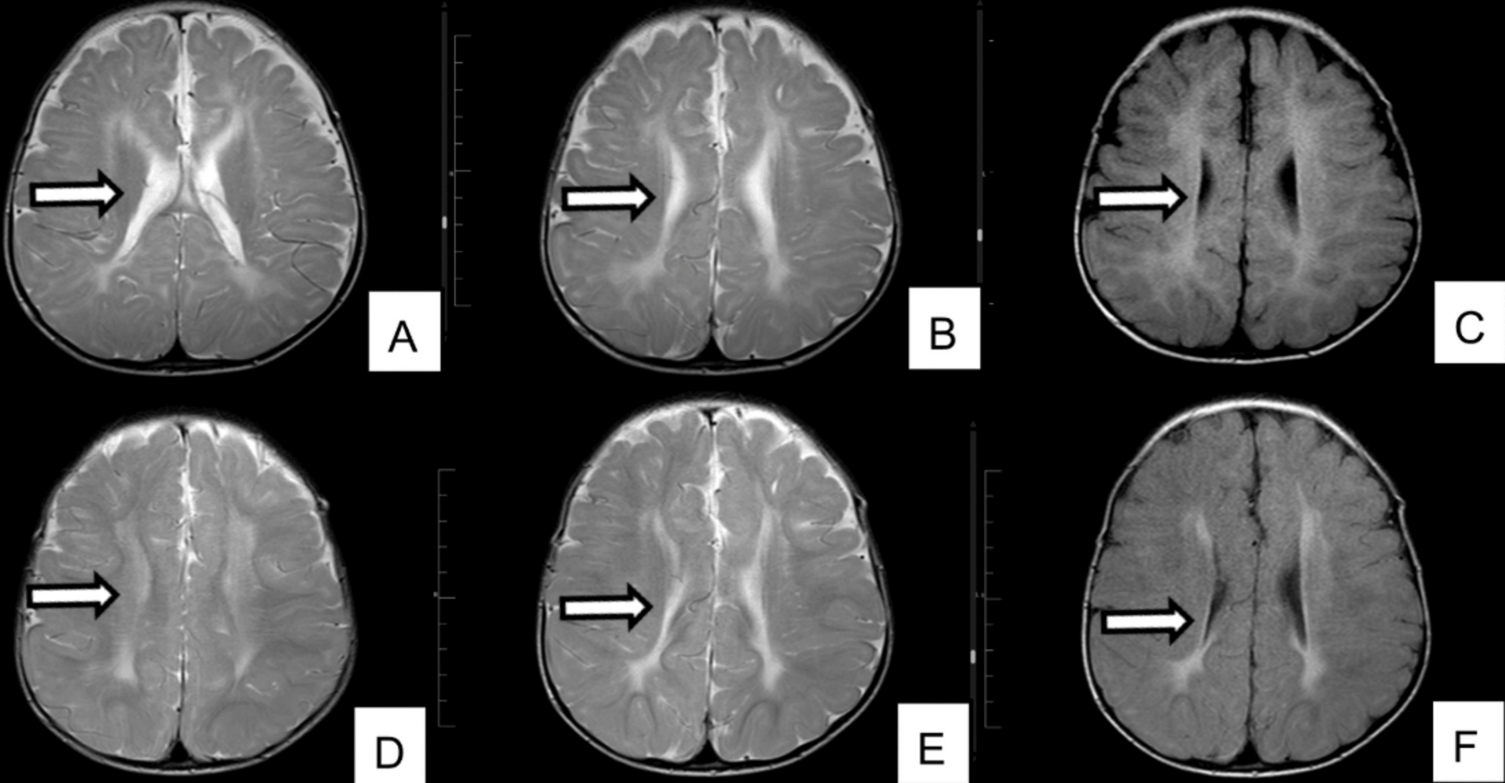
Brain MRI of a patient with mucolipidosis type IV obtained at the age of 15 months (A-C) and repeated at the age of two years (D-F) (A) Adjacent axial T2-weighted image confirming persistent periventricular T2 hyperintensity (arrow) and reduced white matter volume without cystic degeneration or basal ganglia signal abnormality. (B) Axial T2-weighted image demonstrating bilateral symmetric hyperintense signal within the periventricular white matter (arrow), most prominent along the anterior and posterior limbs of the internal capsule. Diffuse thinning of the corpus callosum is evident. (C) Axial T2-weighted fluid-attenuated inversion recovery (FLAIR) image demonstrating bilateral symmetric periventricular white-matter hyperintensity greatest along the anterior and posterior limbs of the internal capsule (arrow), with diffuse thinning of the corpus callosum; ventricles normal. (D) Adjacent axial T2-weighted image showing continued diffuse thinning of the corpus callosum (arrow) without hydrocephalus or cystic periventricular change. (E) Axial T2-weighted image demonstrating bilateral symmetric periventricular hyperintensities (arrow) that appear more prominent, consistent with delayed myelination/hypomyelination. (F) Axial T2-weighted FLAIR image showing persistent bilateral periventricular white-matter hyperintensity (arrow) and reduced white matter volume without cystic change; corpus callosum thinning persists.
